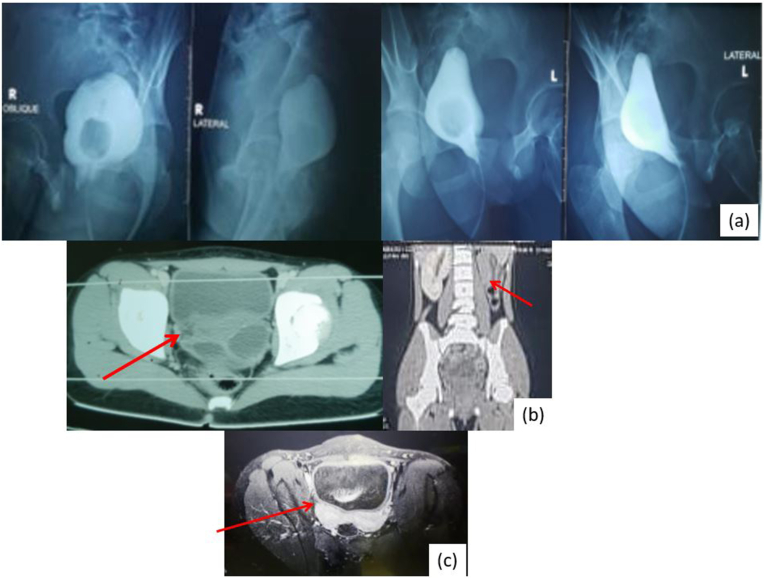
Radiologic Imaging of the patient a. Urethrocystography, b. MSCT of Abdomen and Pelvis, c. MRI Abdomen Pelvis.
